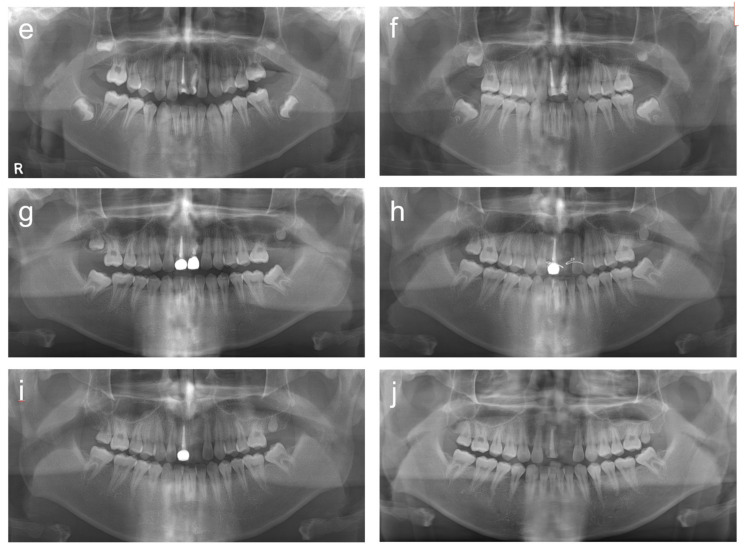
Panoramic radiographs of patient 1. (a) Panoramic radiograph at age 8 years 4 months. (b) Panoramic radiograph at age 9 years 5 months. (c) Panoramic radiograph at age 9 years 7 months. (d) Panoramic radiograph at age 10 years 5 months. (e) Panoramic radiograph at age 11 years 3 months. (f) Panoramic radiograph at age 13 years 2 months. (g) Panoramic radiograph at age 15 years 5 months. (h) Panoramic radiograph at age 16 years 7 months. (i) Panoramic radiograph at age 17 years 9 months. (j) Panoramic radiograph at age 19 years 1 month.
